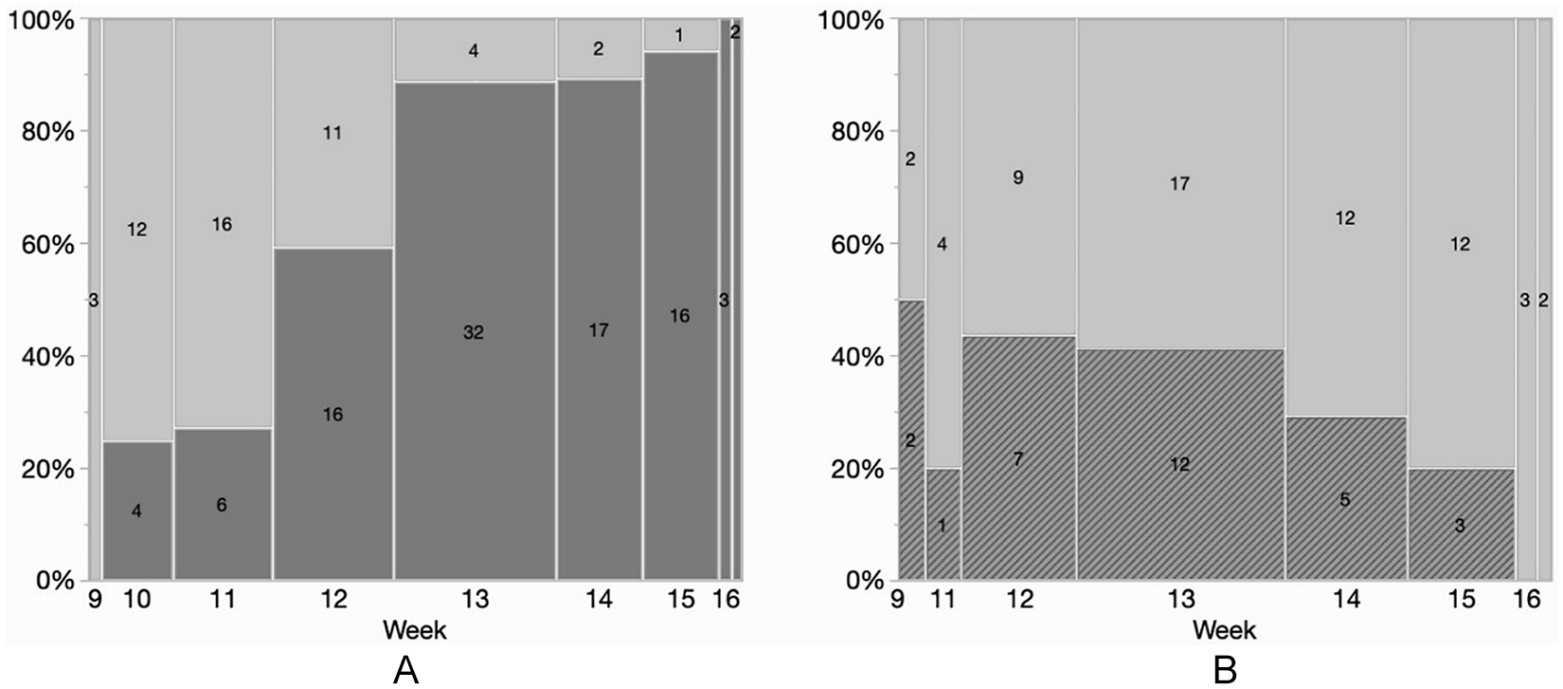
CDUS exams from week 9 (starting on February 24) to week 17 (ending on April 27). (Panel A) Number of patients screened with CDUS (dark gray). (Panel B) Number of positive (dark gray, line pattern) versus negative (light gray) CDUS. *Note.* CDUS = Complete Duplex Ultrasound.
